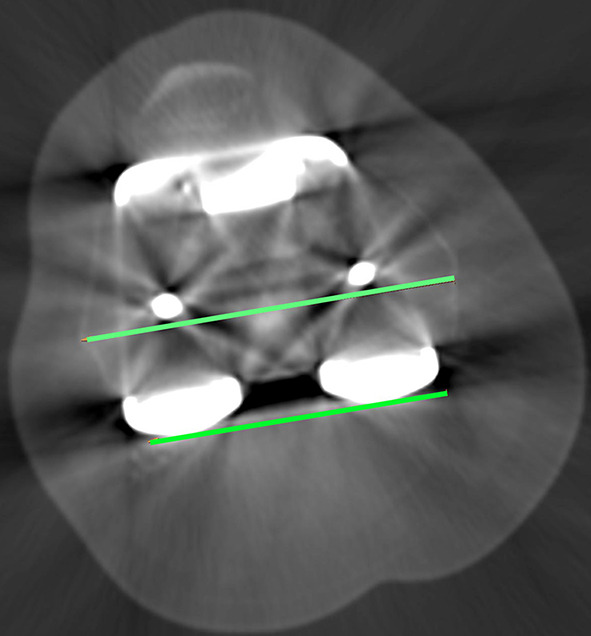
The Berger’s femoral component rotational angle (BFA). Axial CT image showing measurement of the rotation of the femoral component using the surgical transepicondylar axis and the posterior femoral condylar axis. The surgical transepicondylar axis is a line drawn between the lateral epicondylar prominence and the medial sulcus of the medial epicondyle in a slice where the two landmarks were visible. The posterior femoral condylar axis is a line drawn tangential to the posterior surface of the two condyles of the TKA femoral component
